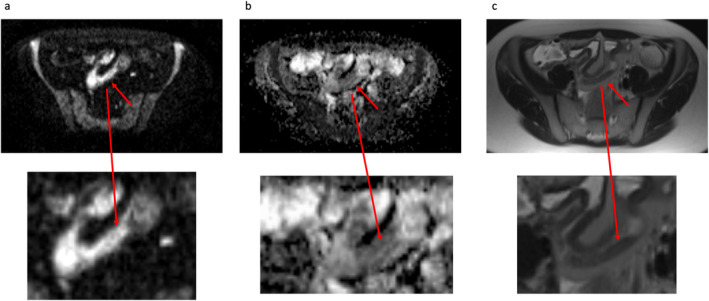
DWI in active CD in the terminal ileum. (a) axial DWI image (*b*‐value = 600 seconds/mm^2^) with increased signal intensity in in the abnormal ileum (red arrow); (b) the ADC_0‐600_ map demonstrates corresponding low ADC in the abnormal ileum in keeping with restriction of diffusion; (c) conventional T2 HASTE image for reference demonstrating the thickened, inflamed ileum. Corresponding magnified images of the abnormality are displayed underneath each image. DWI = diffusion‐weighted imaging; CD = Crohn's disease; ADC = apparent diffusion coefficient; HASTE = half‐Fourier acquisition single‐shot turbo spin‐echo.
